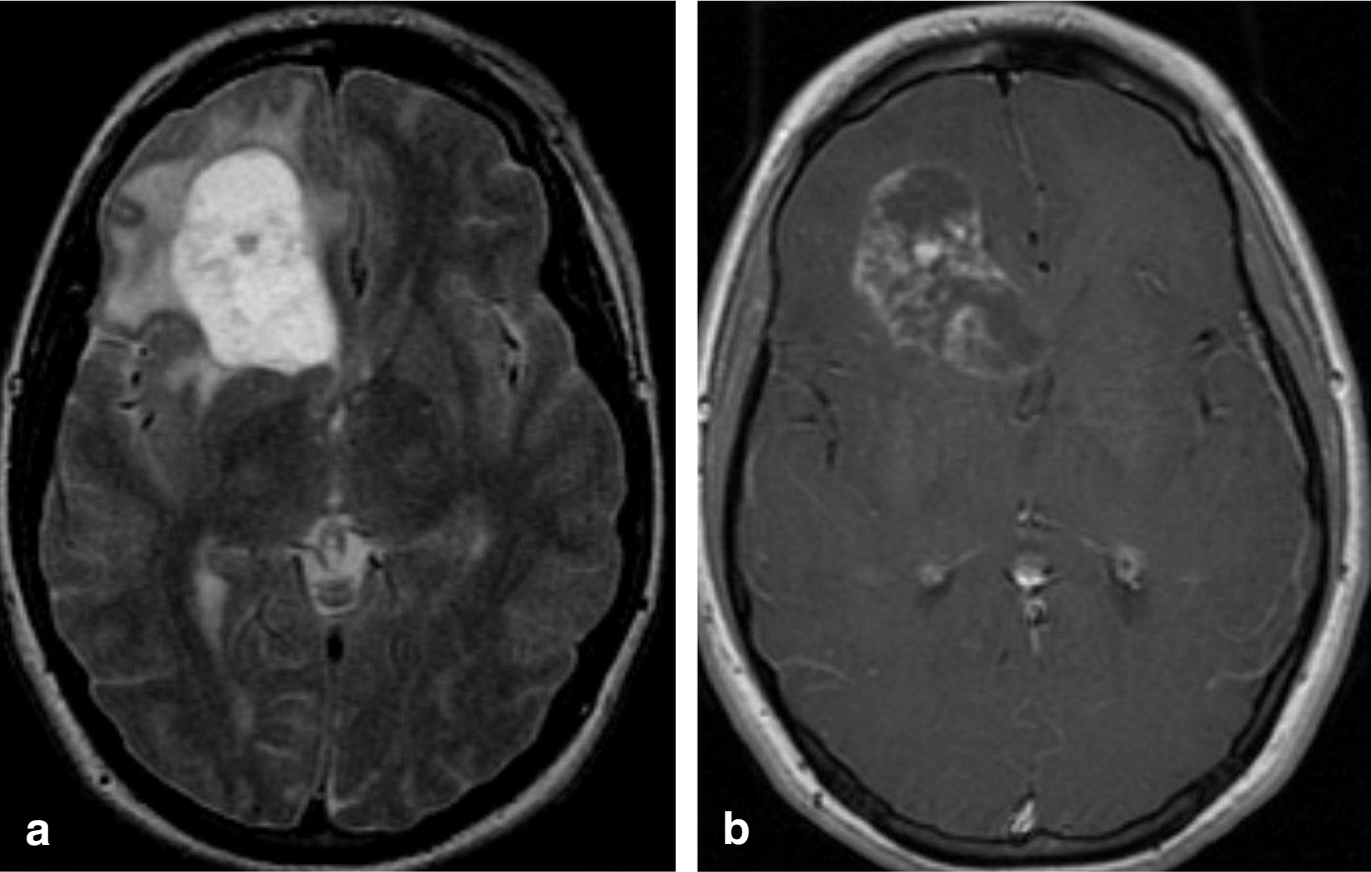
Papillary glioneuronal tumor (PGNT) in a 17-year-old female who presented with a seizure. Axial T2-weighted (a) and T1-weighted contrast-enhanced (b) MR images demonstrate a heterogeneous, predominantly T2-hyperintense right frontal lobe mass with nodular and stippled internal enhancement. Note the moderate degree of surrounding vasogenic edema
